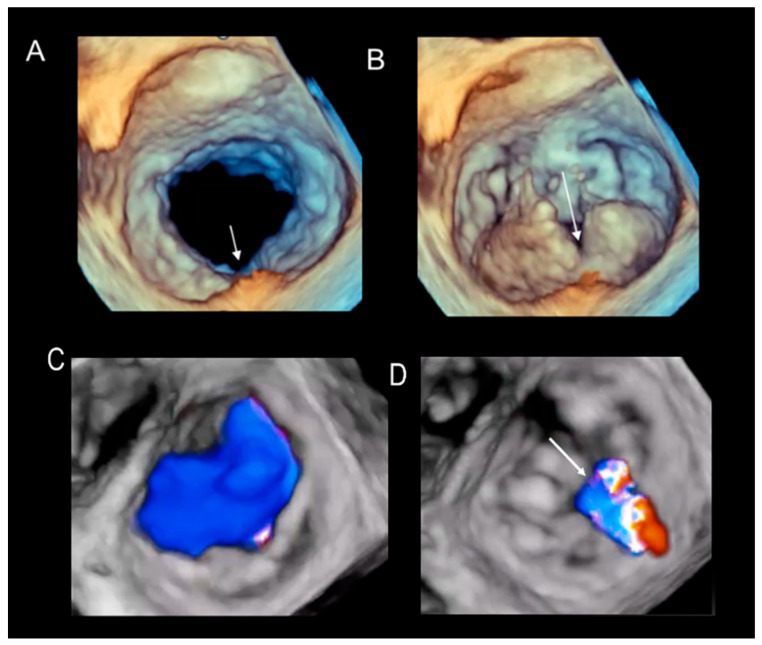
(A,B) Deep cleft (arrow) separating a large P2 into two halves, displayed in diastole (A) and systole (B). (C,D) The same patient using 3D TEE color Doppler. The images show that, in systole (D), the regurgitant jet flows across the cleft.
